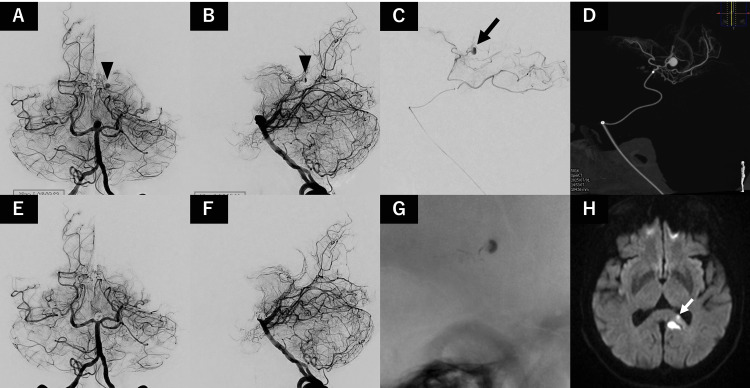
Endovascular treatment of the pseudoaneurysm Left vertebral artery angiography performed one month after the initial hemorrhage during treatment of the basilar tip aneurysm. Pre-treatment delayed arterial phase images are shown in the anteroposterior view (A) and lateral view (B), demonstrating a pseudoaneurysm arising from the splenial artery (black arrowheads). Selective angiography via a microcatheter (C) and cone-beam computed tomography (D) were performed to delineate the precise location of the pseudoaneurysm (arrow). Post-treatment angiography in the anteroposterior (E) and lateral (F) views demonstrates complete occlusion of the pseudoaneurysm with preservation of adjacent branches and near-complete occlusion of the basilar tip aneurysm. A lateral radiograph (G) demonstrates the glue cast, showing adequate penetration into the pseudoaneurysm. Postoperative diffusion-weighted magnetic resonance imaging (H) demonstrates a small spotty hyperintensity adjacent to the hemorrhagic lesion (white arrow).
